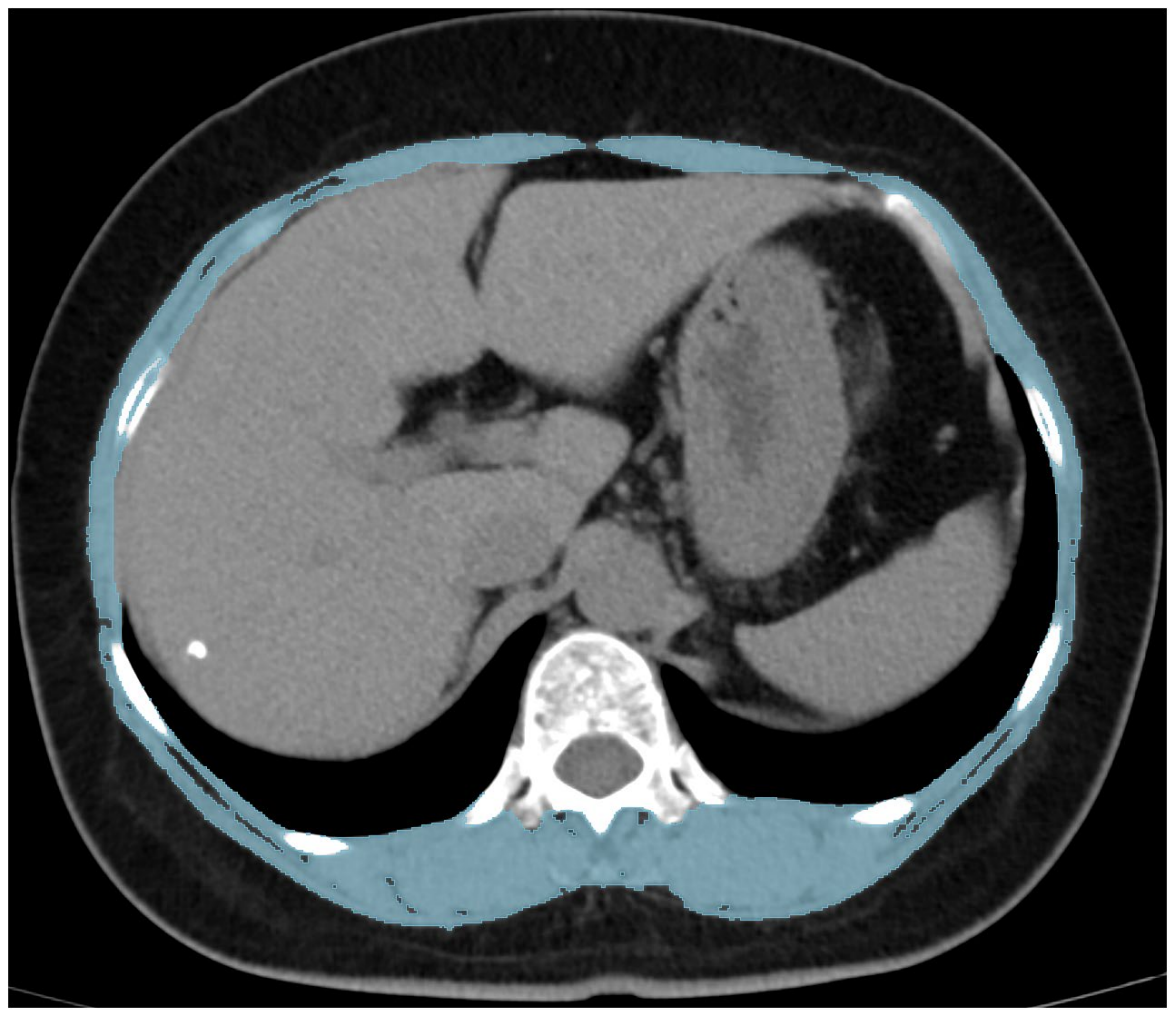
Measurement of the skeletal muscle index using computed tomography at the T12 level. T12 the 12th thoracic vertebra.
